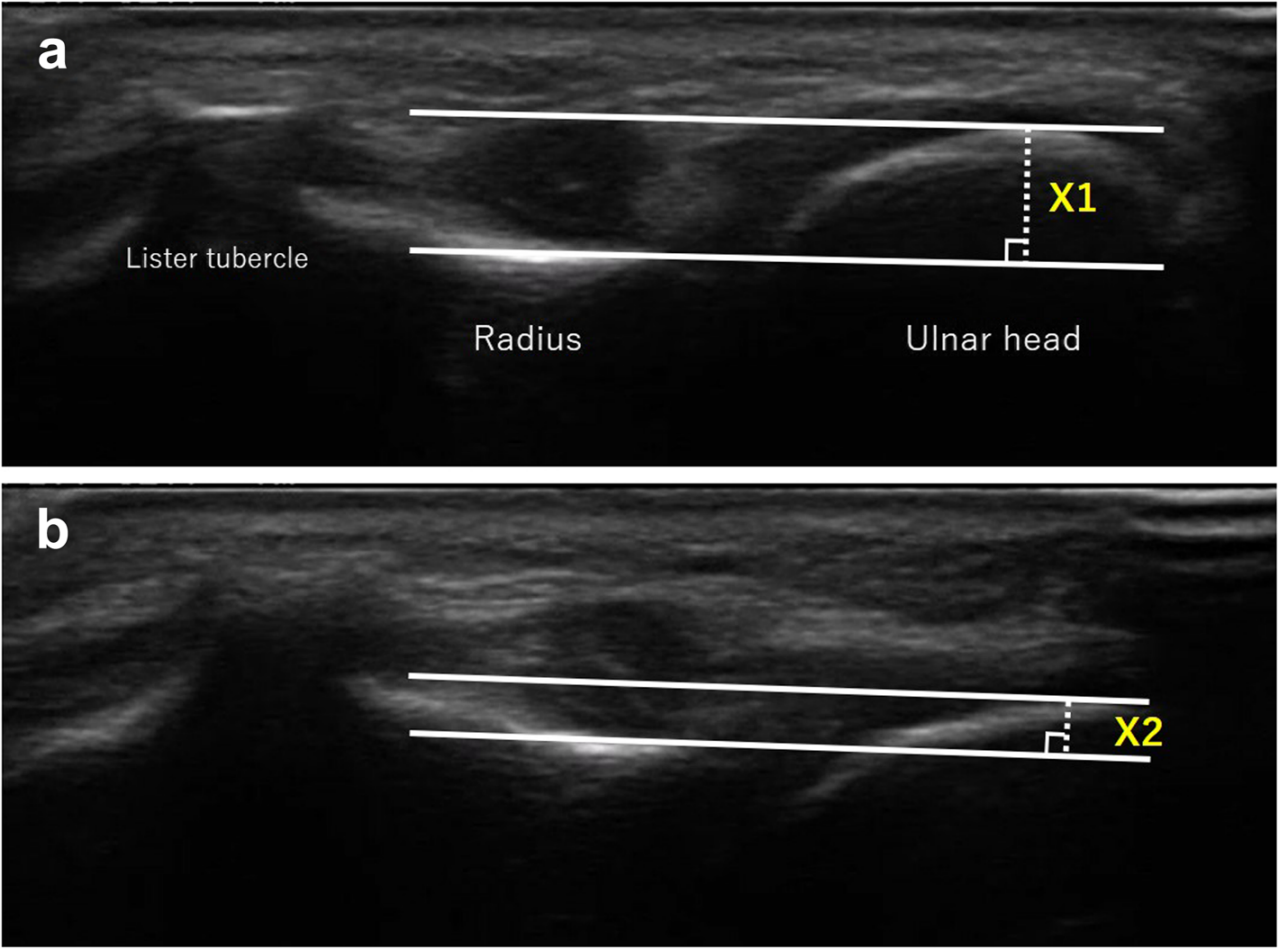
The ultrasonography of the distal radioulnar joint. a Before the compression of the ulnar head. b After the compression of the ulnar head. X1: distance between the dorsal surfaces of the ulnar head and the radius before compression. X2: distance between the dorsal surfaces of the ulnar head and the radius after compression. X1-X2: the volar translation distance of the ulnar head
